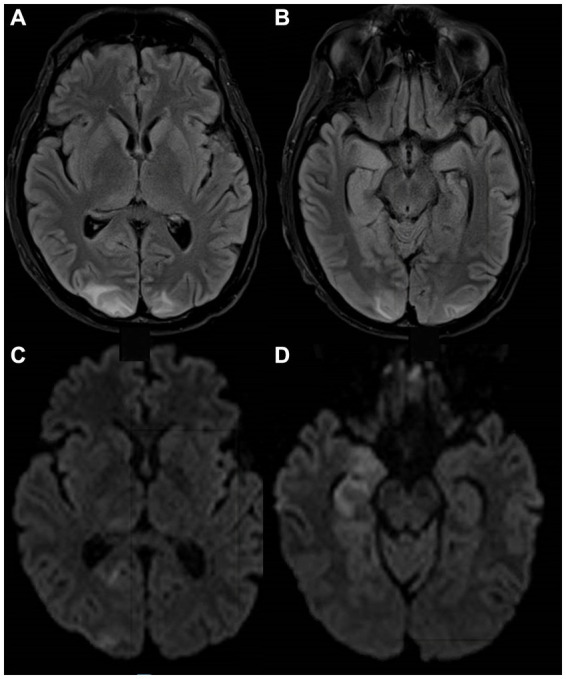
MRI images from a patient with NEMO deficiency and MAC infection. A 40-year-old man with NEMO deficiency and disseminated MAC infection. Images were obtained following a generalized seizure and show T2-FLAIR hyperintense signal in the bilateral occipital lobes (A) and the hippocampi (B), initially considered to be posterior reversible encephalopathy syndrome vs. a post-ictal etiology due to the atypical distribution and restriction of some lesions on DWI (C,D), but a post-mortem analysis of the brain showed microglial activation and nodules in the hippocampi, with tissue culture positive for MAC. DWI, diffusion-weighted imaging; FLAIR, fluid-attenuated inversion recovery; MAC, *Mycobacterium avium complex*; NEMO, Nuclear factor-kappa B Essential Modulator; PRES, posterior reversible encephalopathy syndrome.
